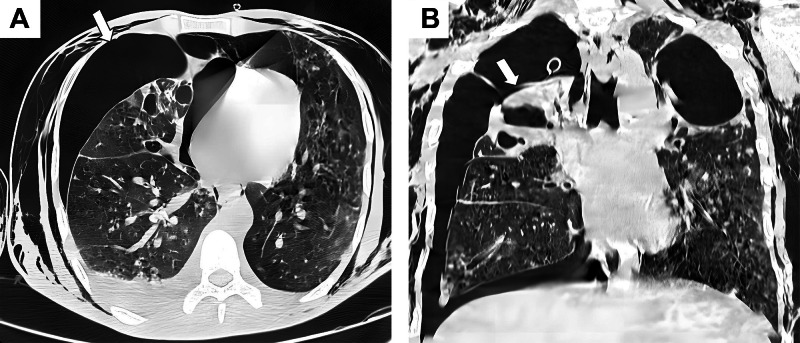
(A) Axial chest CT scan (lung window) obtained after chest drainage, demonstrating marked regression of the right pneumothorax (white arrow) and partial re-expansion of the right lung. (B) Coronal chest CT scan (lung window) obtained after chest drainage showing marked regression of the right pneumothorax (white arrow) and partial re-expansion of the right lung. The chest tube is seen in place. Note the presence of residual bullous and bronchiectatic changes in the right lung parenchyma and fibrotic alterations in the left lung, consistent with chronic postinfectious sequelae.
